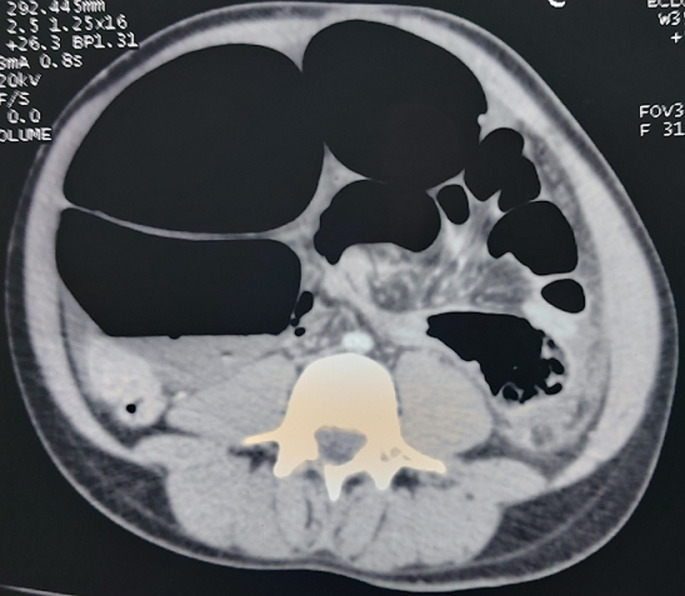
the abdominal CT scan revealed a whirl sing and significant distension of the sigmoid loop, measuring 12 cm in diameter with parietal thinning
